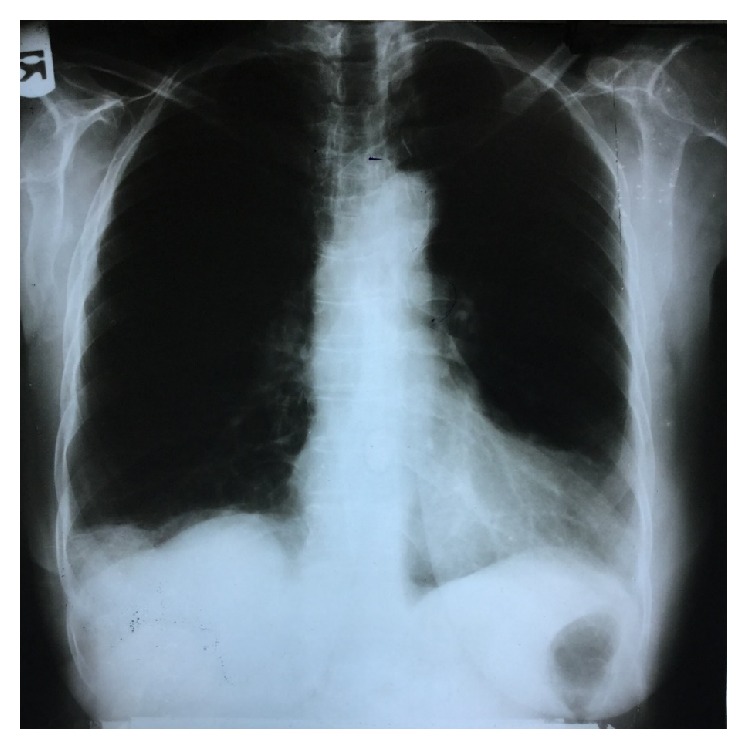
X-ray chest showing right diaphragmatic hernia.
